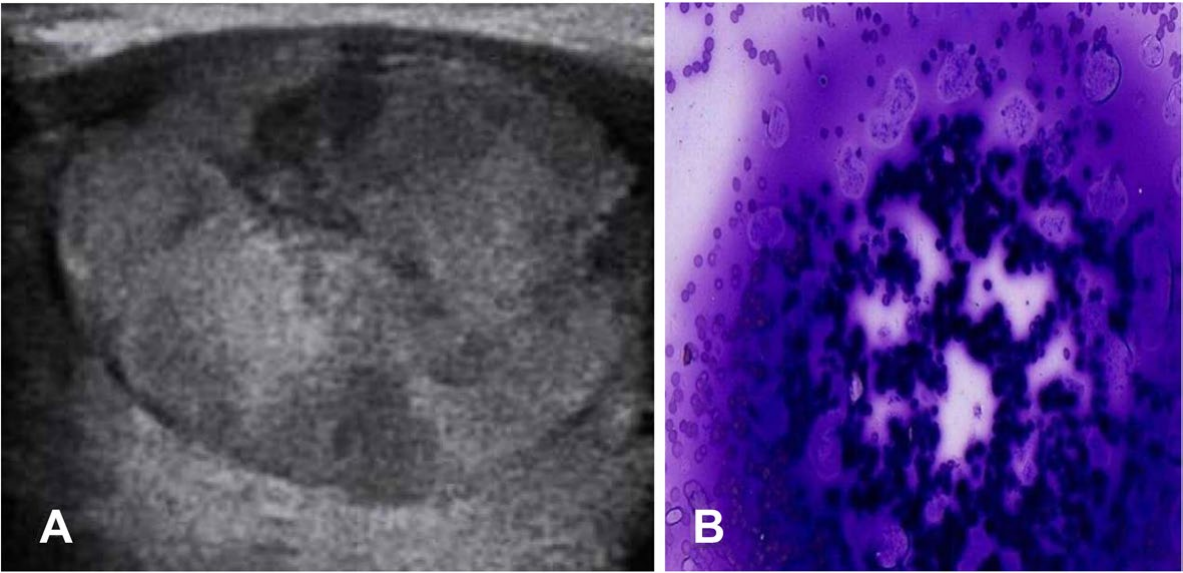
A Transverse sonogram of a well-defined wider than taller isoechoic solid nodule in the upper outer quadrant of the left lobe in 24-year-old women. It was classified as solid (composition score of 2), With 1 more point for iso-echogenicity and none in other categories, its total point was 3 (TR3). B Cytology result showing abundant thick colloid benign follicular cells (diff-quick, × 40) (category II bethesda system)
